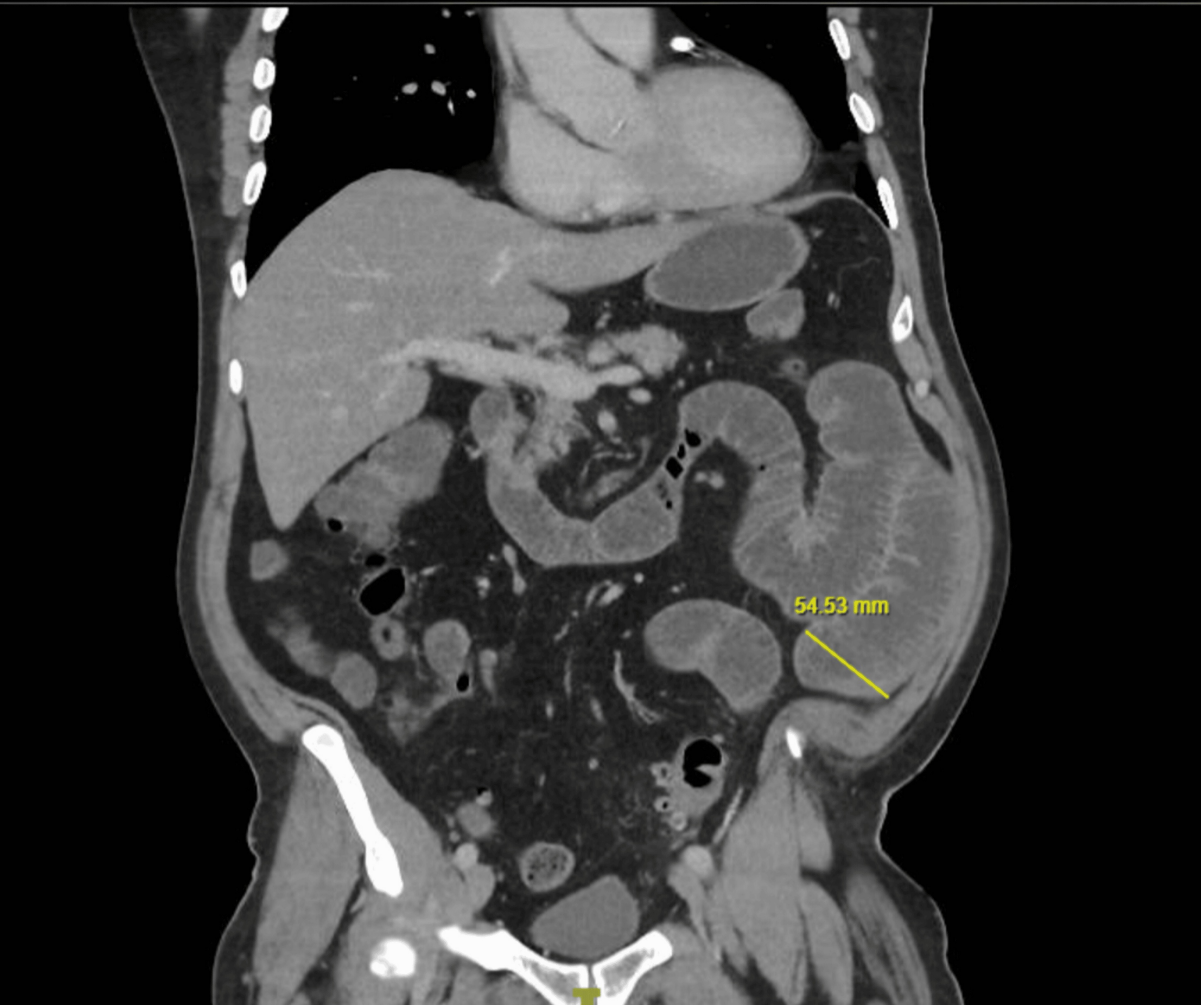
Coronal view of contrast-enhanced CT of the abdomen Dilated small bowel loops (up to 54 mm) with mural thickening and enhancement are seen adjacent to the sigmoid colon, consistent with reactive enteritis.
